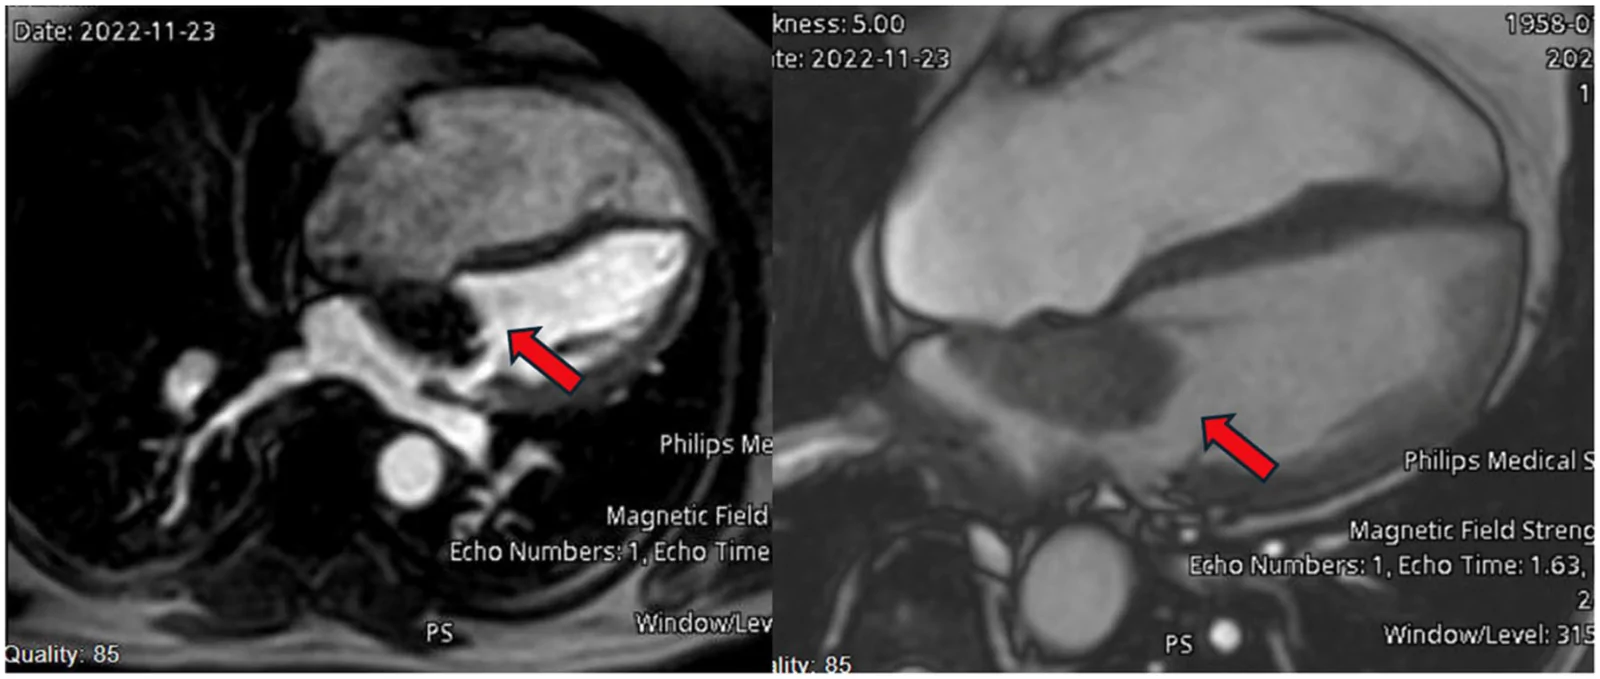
MRI images T1,T2-weighted sequences. Showing dilated left atrium with an oblong to broad-based lobulated lesion (red arrow) within the left atrial cavity with mild lobulation and attached to the interatrial septum near the base and abutting the mitral valve leaflet orifice, it measures around 4.6 cm × 2 cm in its maximum dimension. The mass has isointense signal intensity on the T1-weighted sequences, iso to mildly hyperintense on the T2-weighted sequence, the perfusion images shows no vascularity.
